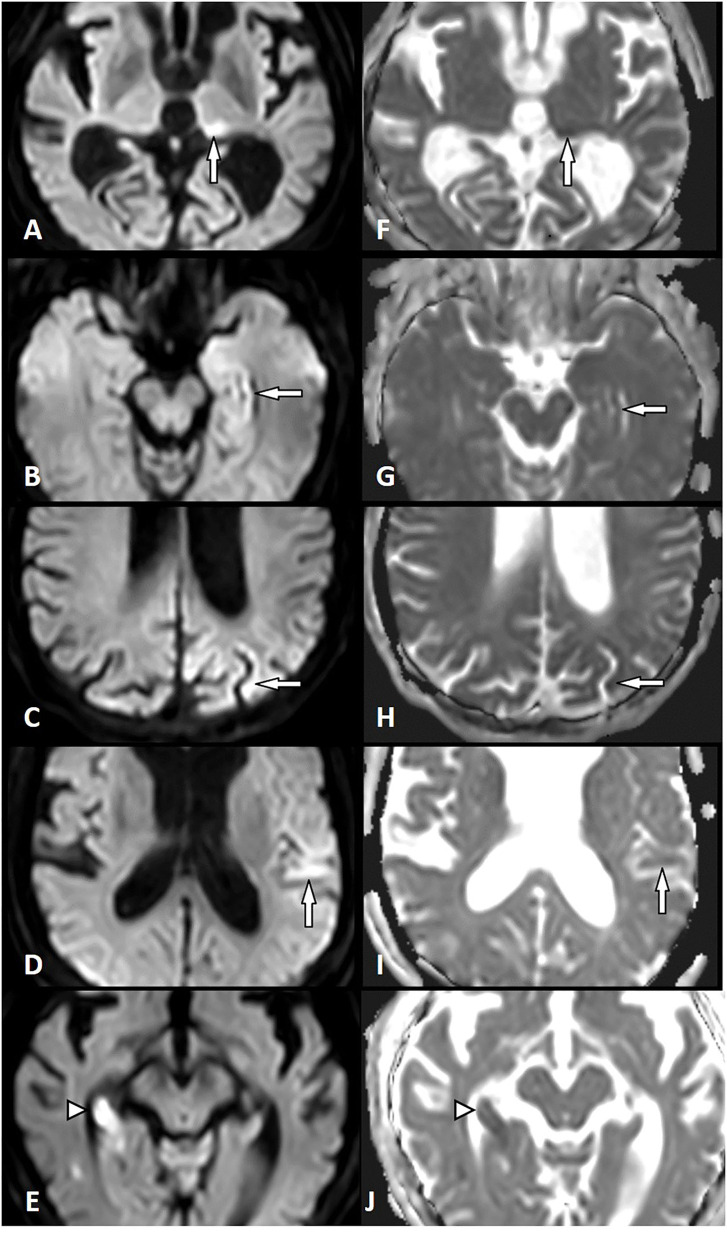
Diffusion restriction in status epilepticus (SE) and acute ischemic stroke (AIS). Left column: Diffusion-weighted imaging (DWI). Right column: Apparent diffusion coefficient (ADC). SE-related diffusion restriction in the left pulvinar (A,F), in the left hippocampus (B,G), the left parietal cortex (C,H), and the left temporal cortex (D,I). AIS-related diffusion restriction in the right hippocampus (right posterior cerebral artery territory) (E,J) (white arrow, SE lesion; white arrowhead, AIS lesion).
